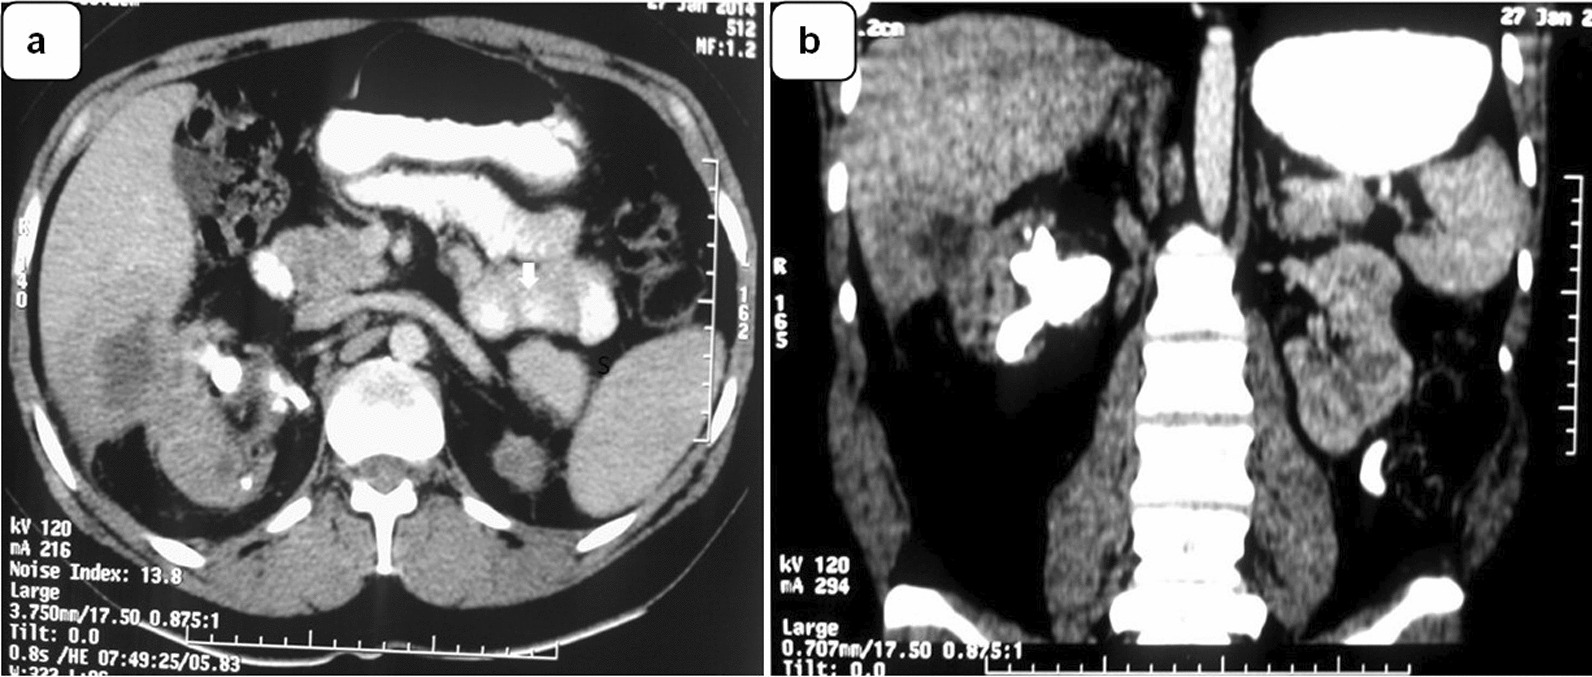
a Transverse CT scan showing the liver abscess surrounded by an area of intermediate density corresponding to the hypoechoic rim seen on ultrasound. b Coronal CT scan shows a staghorn stone in the right kidney and a hepatic abscess most likely located in segment VI of the liver forming a heterogeneous mass involving perirenal fat and the adjacent renal parenchyma
